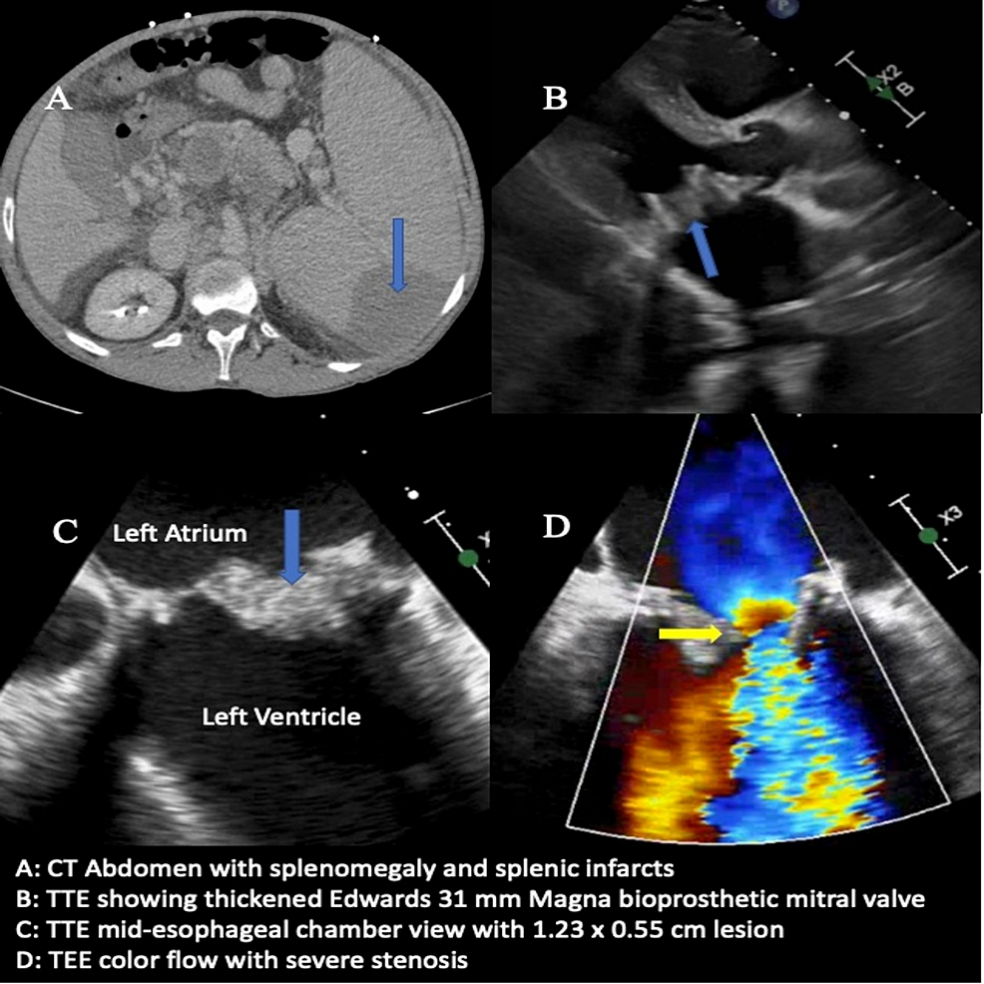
Computed tomography of the abdomen, transthoracic echocardiography, and transesophageal echocardiography findings D: Doppler findings; mitral mean velocity: 2.7 m/sec; mitral mean gradient: 17 mm Hg (severe > 10 mm Hg)
